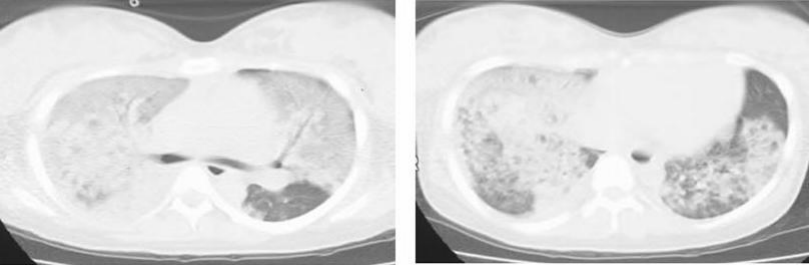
The patient's chest CT prior to admission to the intensive care unit. Homogeneous ground-glass opacity was observed in the peripheral field and spread out into the visceral pleura. Severe infiltration along the bronchoalveolar bundle was observed in the hilar portion.
